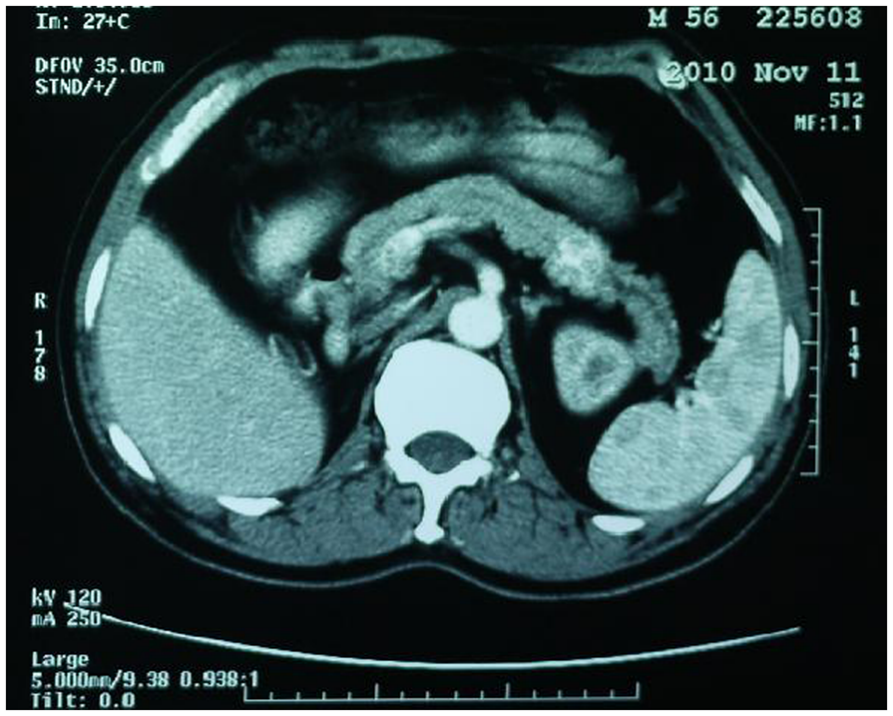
Abdominal computed tomography showed a 2.0 cm enhancing mass in the pancreatic tail. According to CT arterial phase, the pancreatic mass revealed enhancing lesions.
